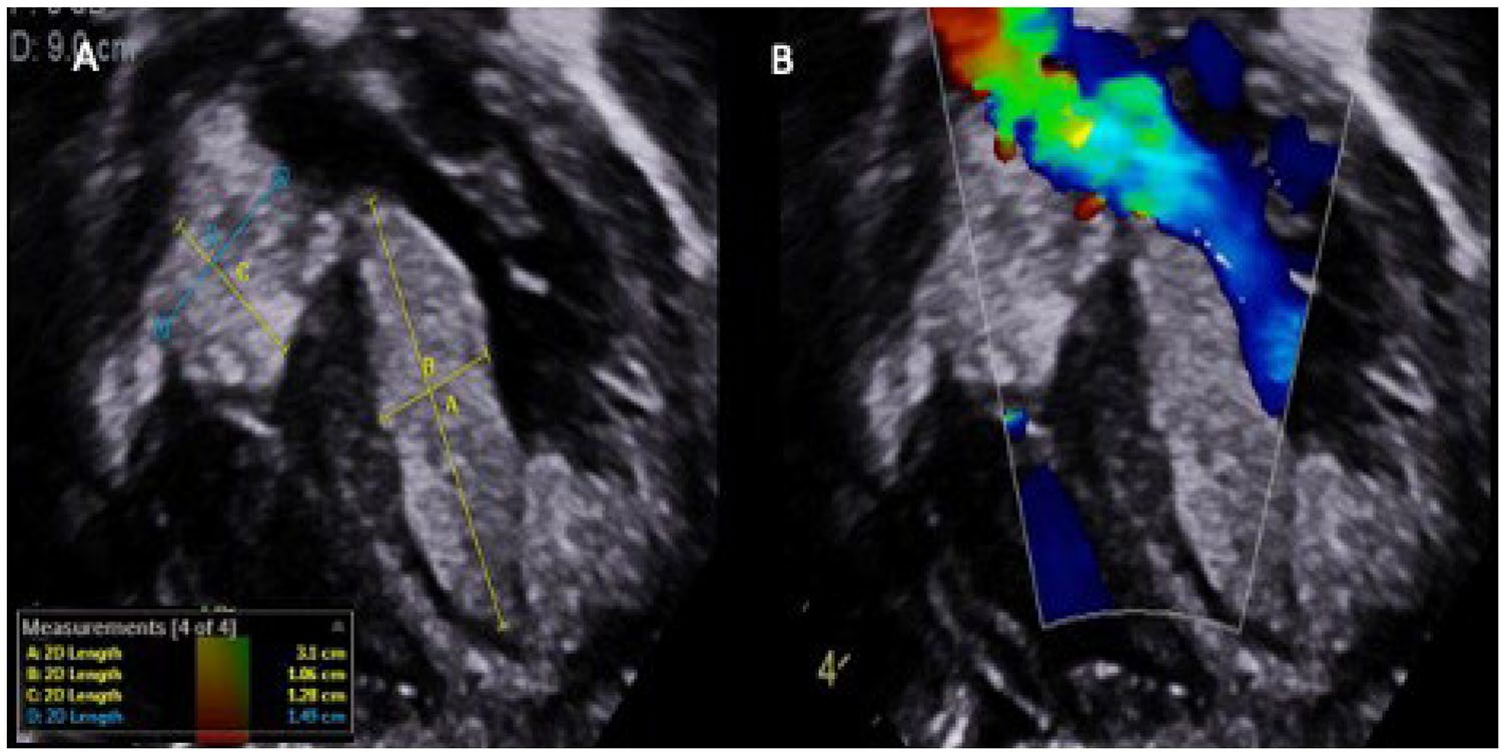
A&B Transthoracic Apical five-chamber (A5C) view with color Doppler compare on initial presentation in Case-5 showing multiple intracardiac rhabdomyomas in the left ventricle (LV) crowding the intraventricular cavity and left ventricular ouflow tract (LVOT) and a large tumour in the right atrium partially obstructing the right ventricular inflow
